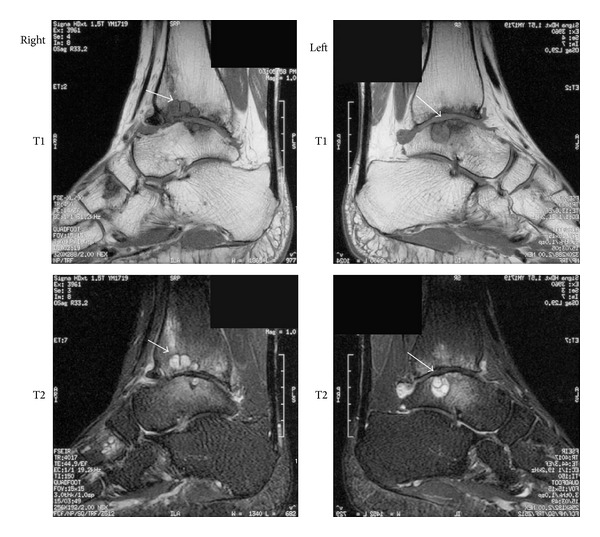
Magnetic resonance imaging (MRI) of both the ankles. Arrows show cystic lesions in both the tibia and talus. Degenerative change is also seen in right cuneiform.
